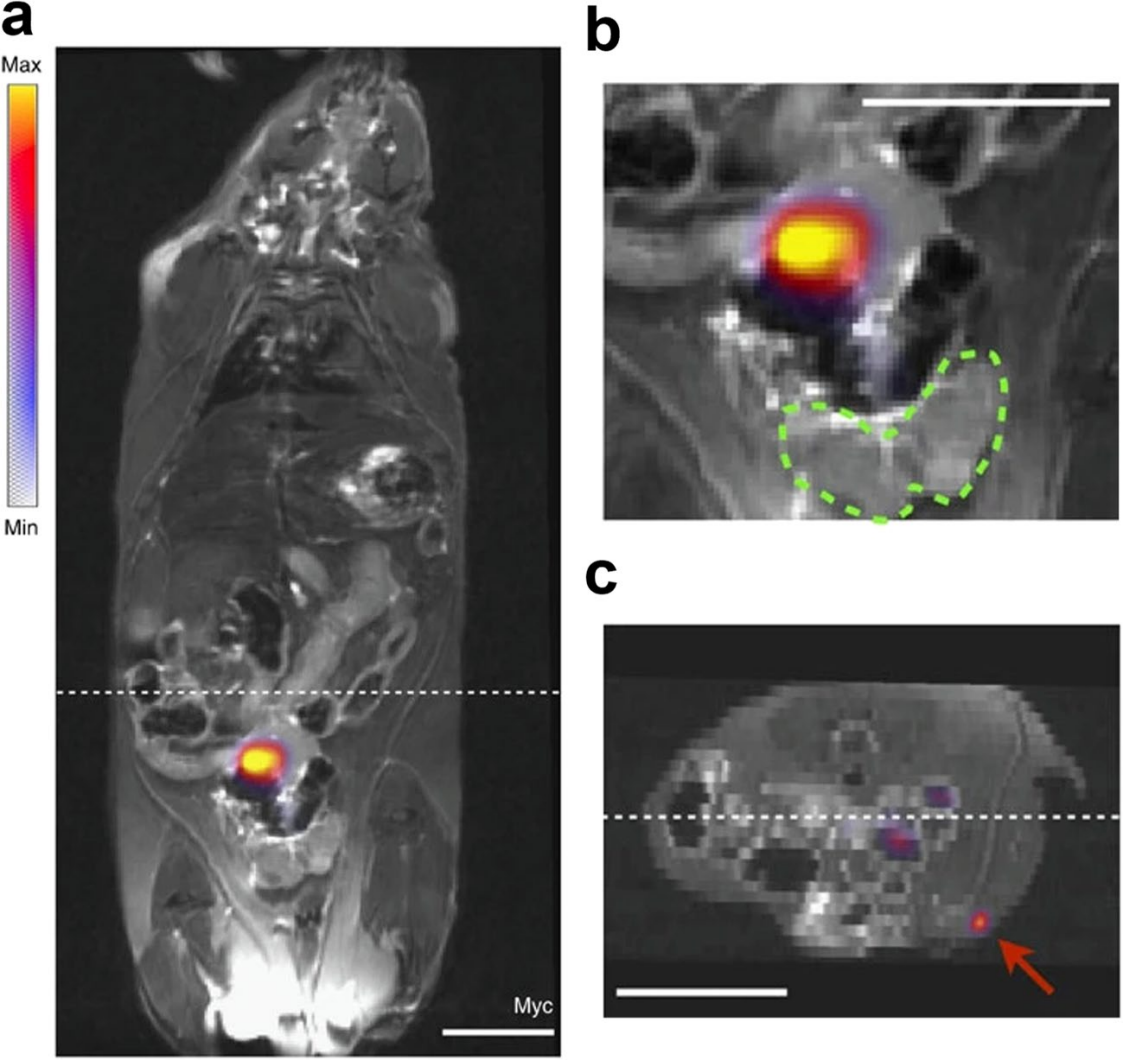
a Whole body coronal PET/MRI fused slice of [89] Zr-ferumoxytol in a draining lymph node, 6 h post-injection. b Magnified prostate region distinctly shows that the draining LN is outside of the prostate organ. The ventral prostate in this slice is delineated by a dashed green outline. c In the reconstructed axial orientation (located at the hashed marks on the coronal image), sensitive PET detection enables visualization of tracer in a more distant draining inguinal node (red arrow). In the clinical context, the detection of a draining LN outside of the pelvic bed would necessitate a widened field of biopsy sampling or therapeutic intervention. All scale bars are 0.8 cm in length. (Adapted with permission from Thorek et al., 2014, ©2014, Nature Communications, published by Springer Nature)
